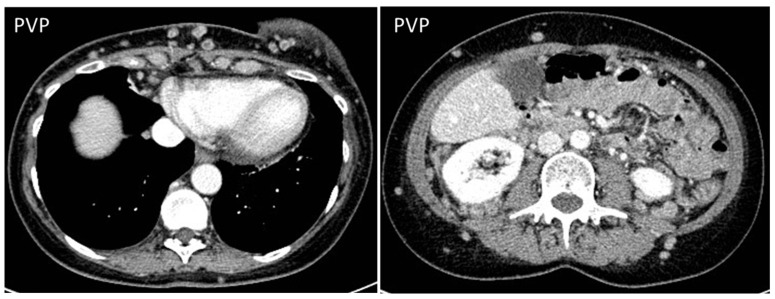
CT on portal venous phase in a woman who recently underwent quadrantectomy for breast cancer shows multiple nodules spreading from the site of the operation to the subcutaneous fat of the anterior and posterior abdominal wall.
